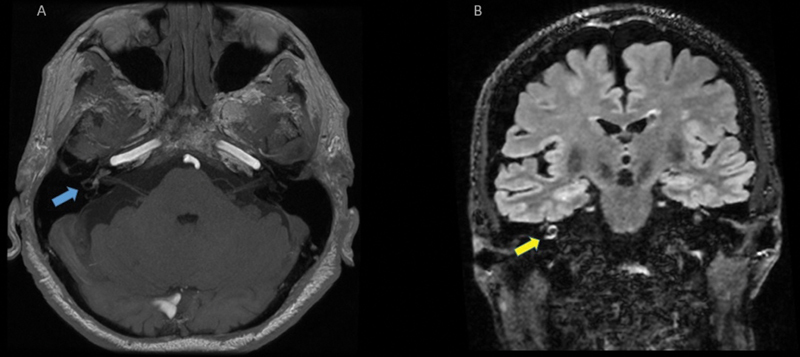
Magnetic resonance imaging (MRI) findings consistent with right labyrinthine hemorrhage. (
